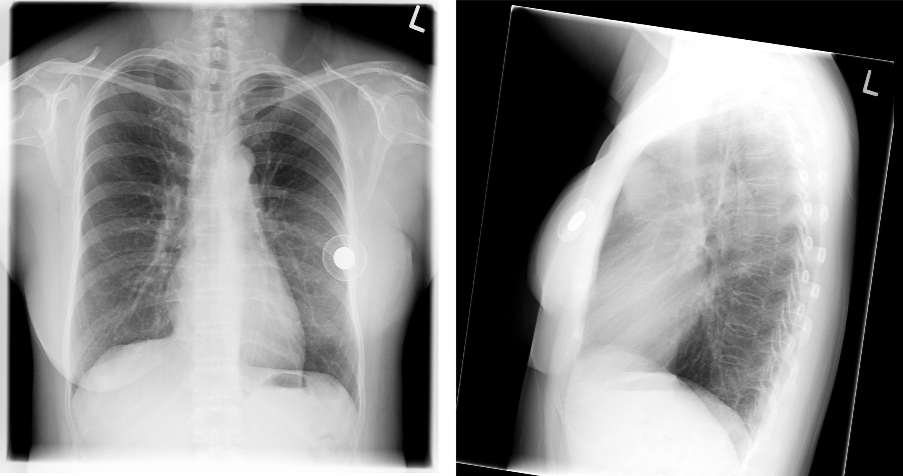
Patient 1. A chest radiograph revealed a dense ring-like object in the region of the left breast, which was the metallic injection port of the left breast tissue expander.
